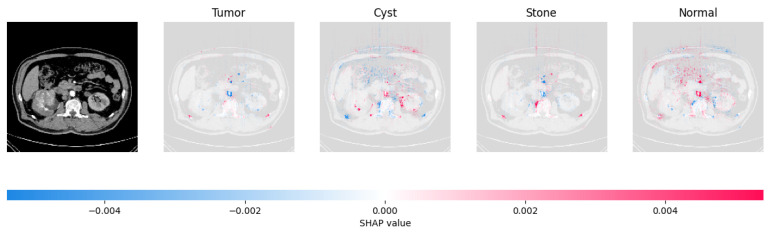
The model found that the CT image is predicted as Normal as the high concentration of red pixels is located in the fourth explanation image.
